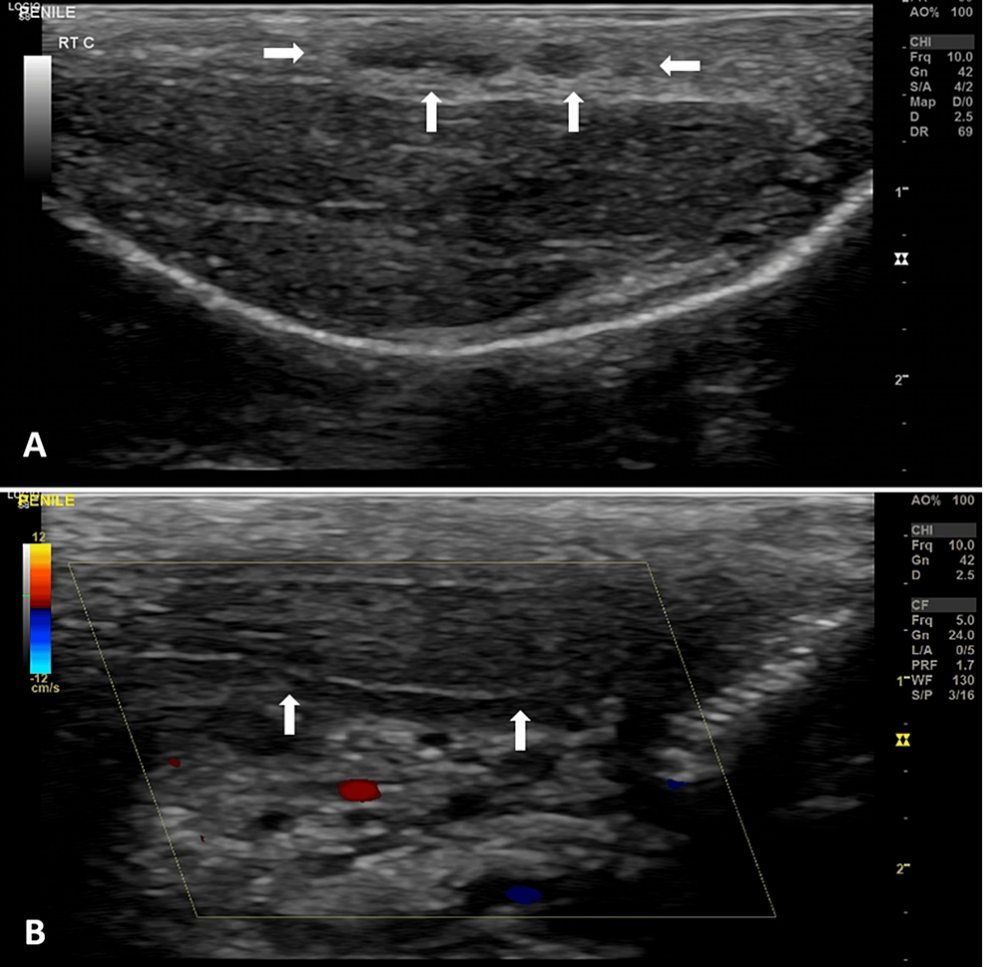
Penile ultrasound: longitudinal section Echogenic thrombus within the superficial dorsal vein of the penis (white arrows in A, B) with no flow on the Penile Color Doppler Ultrasound (white arrow in B).
